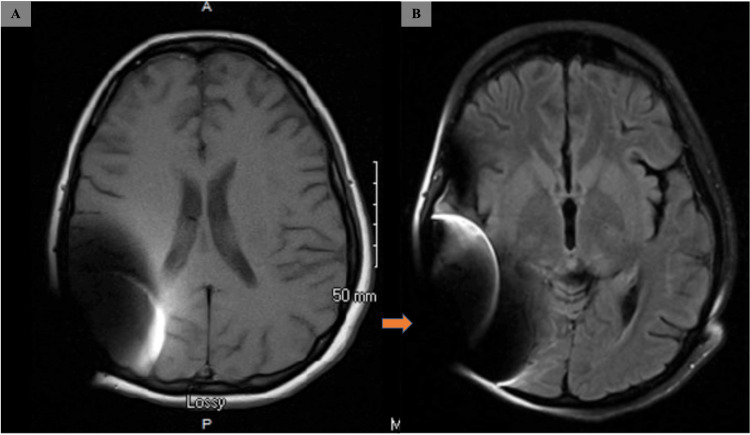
Brain MRI of the patient A: No acute intracranial abnormalities observed on MRI of the brain without contrast; B: Right cerebellum markedly limited secondary to artifact from a cochlear implant (orange arrow)
